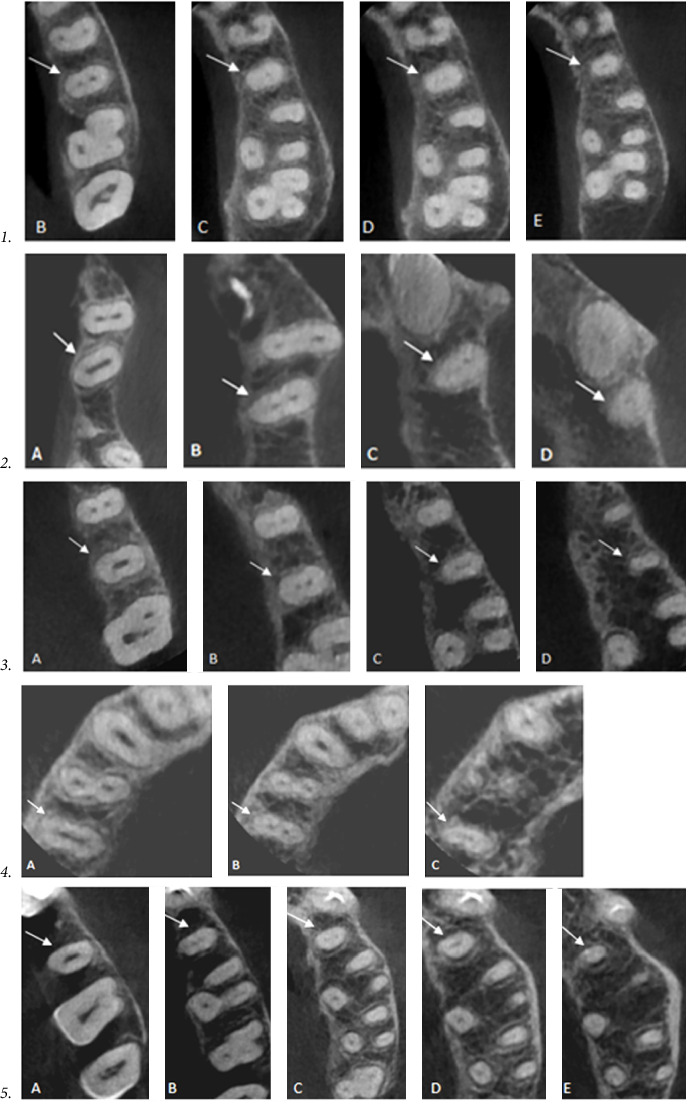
CBCT in the axial plane revealed supplemental and additional canal configurations (at different cross-section from A–E); 1, Gulabivala supplemental canal-configuration type IV (2–1–2–1); Additional types; 2, Sert and Bayirli type (1–2–3–1); 3, Sert and Bayirli type (1–2–3–2); 4, E. Senan type (1–2–3); 5, New canal configuration type (1–3–2–1–2).
